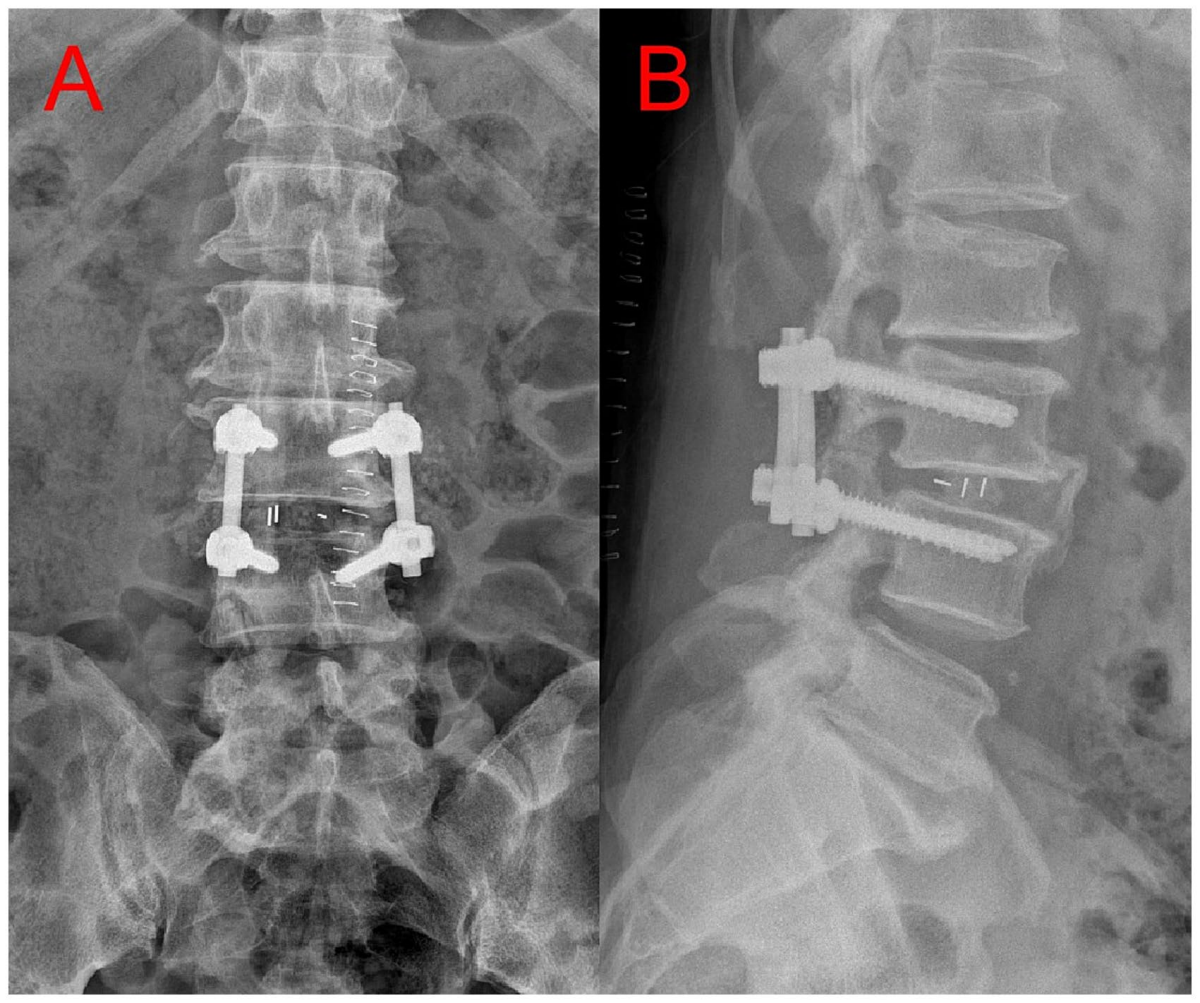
(A,B) Lumbar spine X-ray (1 week postoperatively) indicate adequate decompression with the internal fixation devices positioned at optimal angles.
